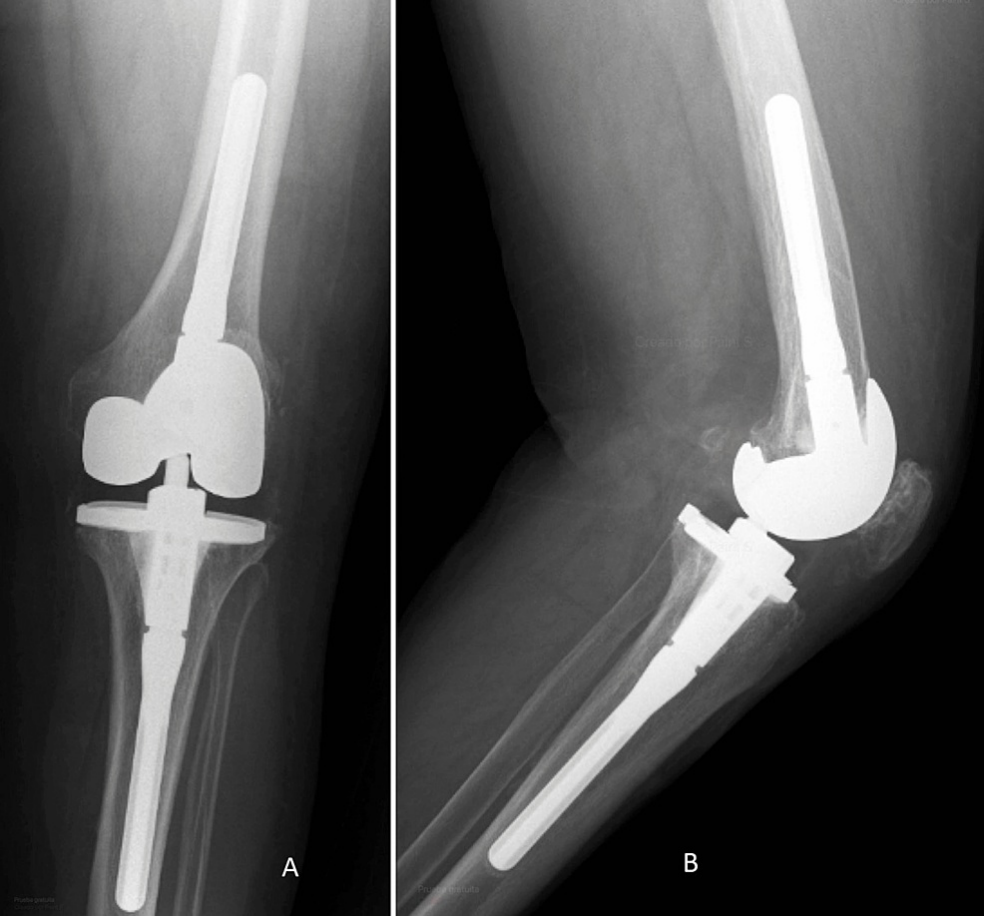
Anteroposterior (A) and lateral (B) radiographs of the revision TKA after the DAIR procedure at one year of follow-up. TKA: total knee arthroplasty; DAIR: debridement, antibiotics, and implant retention.
